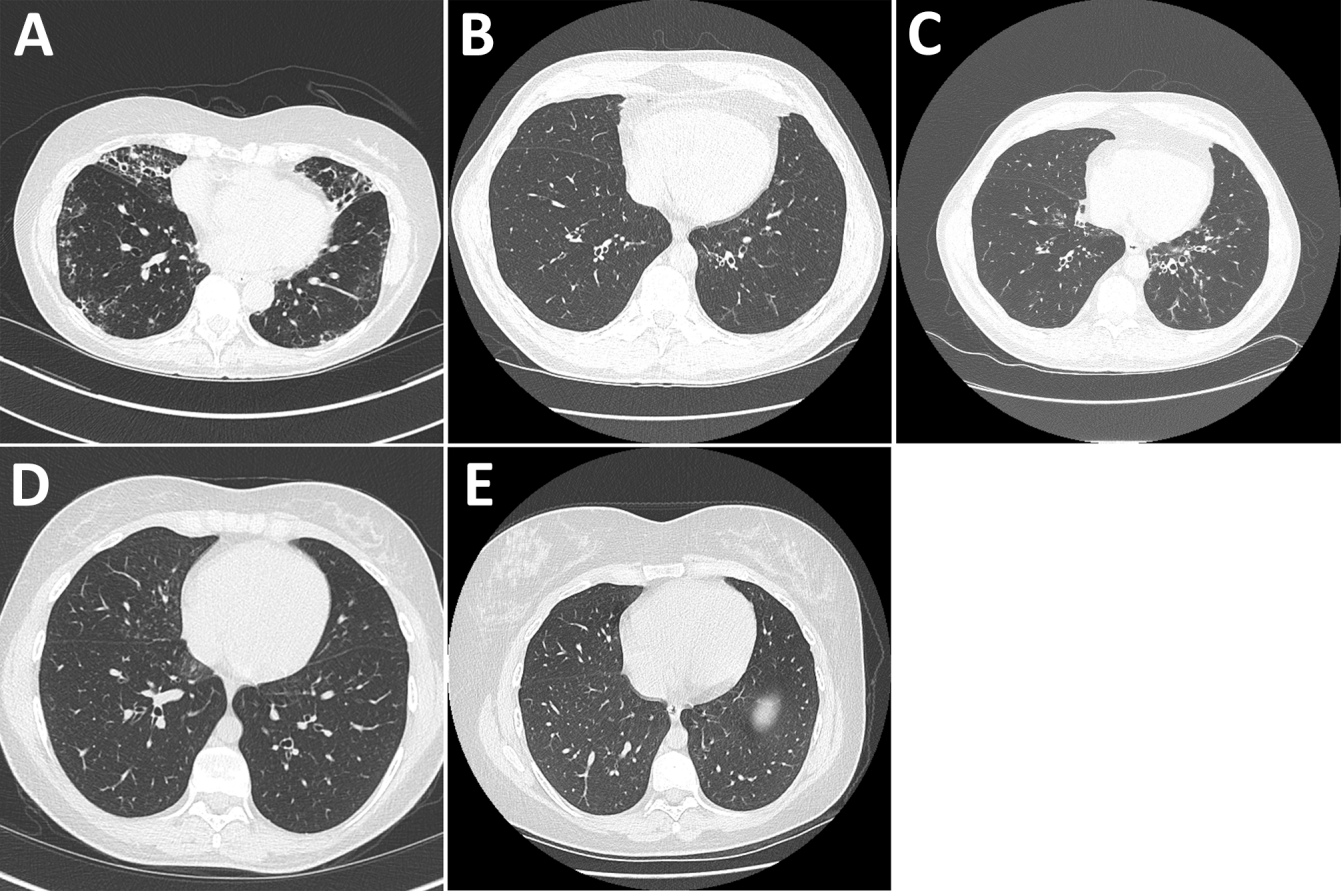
High-resolution chest computed tomography scans from study of *Mycobacterium nebraskense* isolated from patients in Connecticut and Oregon, USA. A) Scan from case 2 in Connecticut showing bronchiectasis in the right middle and lower lobe, left lower lobe, and lingula and tree-in-bud nodularity in the lingula and right and left lower lobes of the lung. B) Scan slice from case 3 in Connecticut through the lower lung lobes showing 1 borderline dilated airway in the left lower lobe, performed in May 2019. C) Scan slice from case 3 at the same anatomic level showing numerous dilated airways and airway wall thickening in the left lower lobe of lung, performed in April 2020 soon after the first isolation of *M. nebraskense*. D) Scan from case 4 in Connecticut showing tree-in-bud nodularity in the right middle and lower lobes of the lung when patient had *M. nebraskense* isolated from her sputum in August 2020. E) Scan from case 4 in Connecticut showing resolution of the tree-in-bud nodularity in the right middle and lower lobes of the lung, performed in December 2022.
